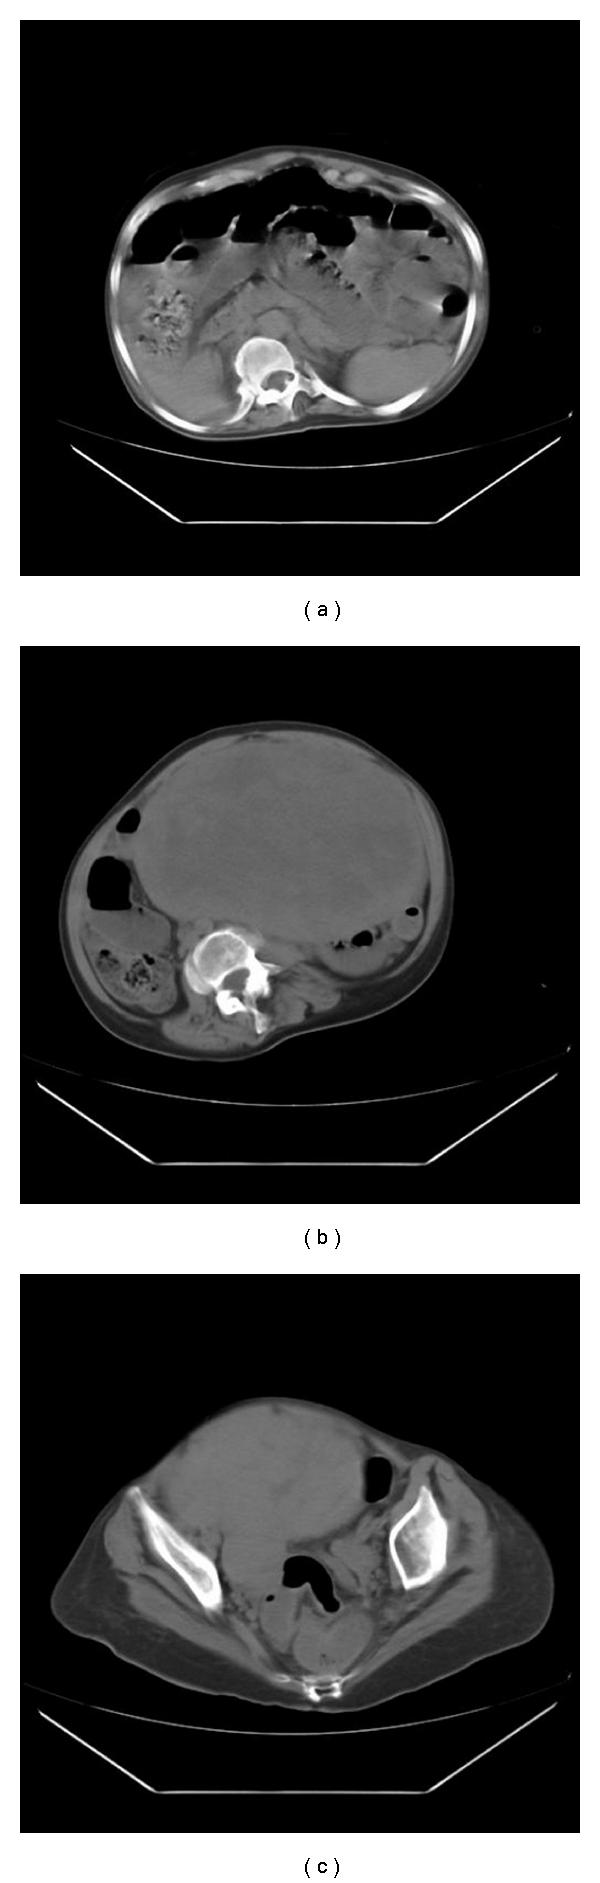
(a–c) MRI images indicating a huge mass occupying the greatest part of the abdominal cavity compressing the intestinal loops.
